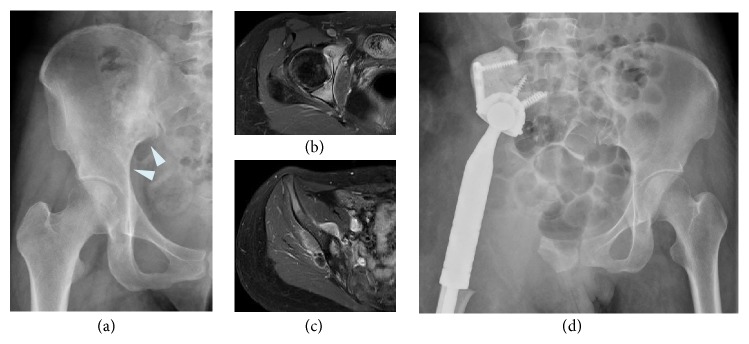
(a) Preoperative anteroposterior radiograph of the pelvis of a 28-year-old female who had osteosarcoma of the acetabulum and ilium. ((b) and (c)) Gadolinium-enhanced axial T1-weighted MRI showing the tumor arising in the acetabulum and ilium. After neoadjuvant chemotherapy, the patient underwent tumor wide resection and endoprosthetic reconstruction with no postoperative major complications. (d) Postoperative radiograph 3 years after endoprosthetic reconstruction.
